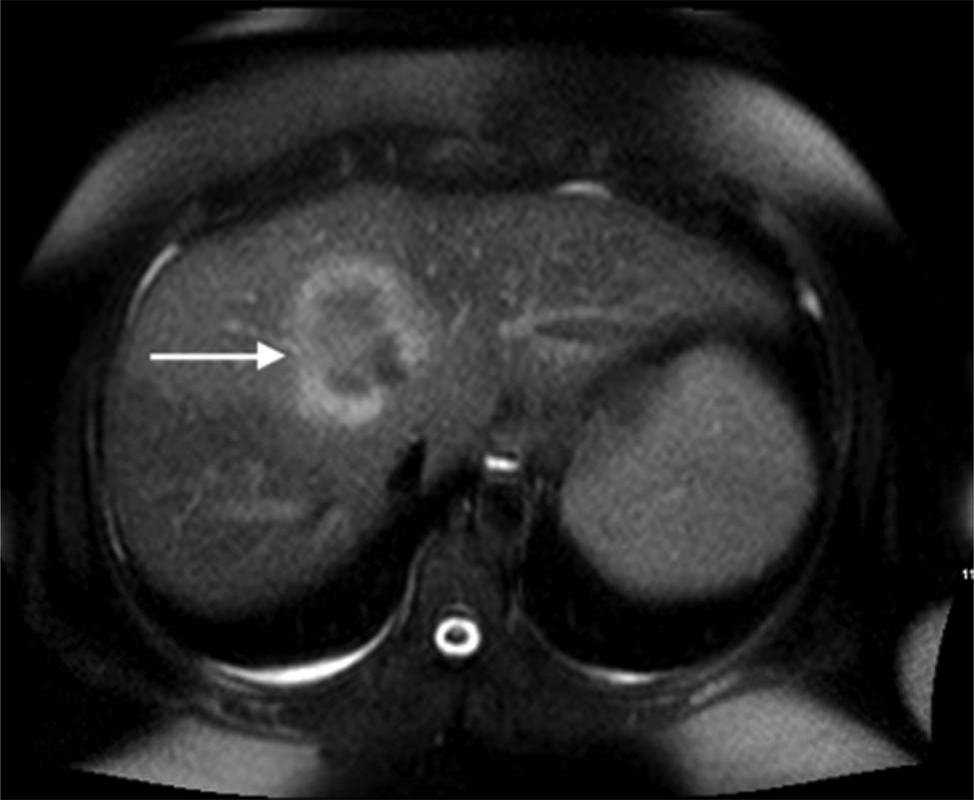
T2 FLAIR-weighted magnetic resonance imaging demonstrating pretreatment 61 by 49 mm hepatic granuloma. FLAIR, fluid-attenuated inversion recovery.
